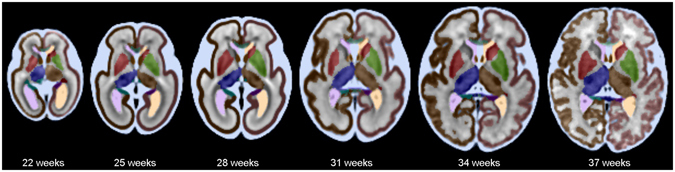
Tissue segmentation and structural labels defined and overlaid on the spatiotemporal fetal brain MRI atlas at six representative GAs: 22, 25, 28, 31, 34, and 37 weeks. The labels visible on these axial sections include the developing white matter, cerebrospinal fluid (CSF), corpus callosum, and left and right gray matter (cortical plate), ventricles, thalami, hippocampi, and lenticular and caudate nuclei.
